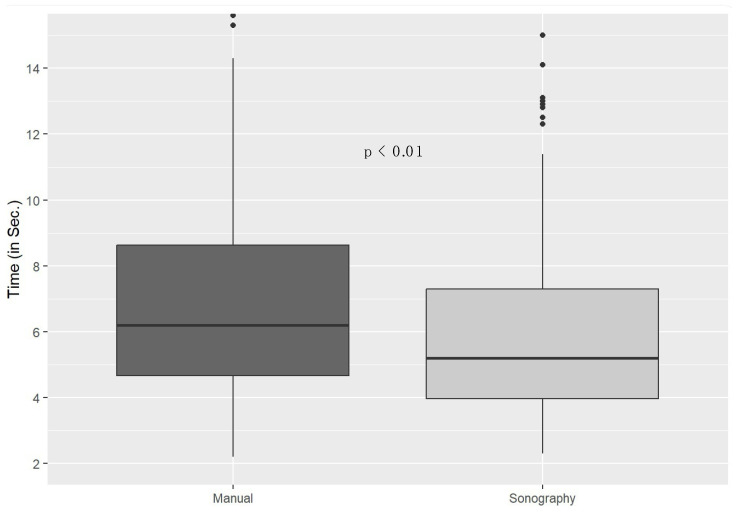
Box plots showing manual and ultrasound pulse check durations.
